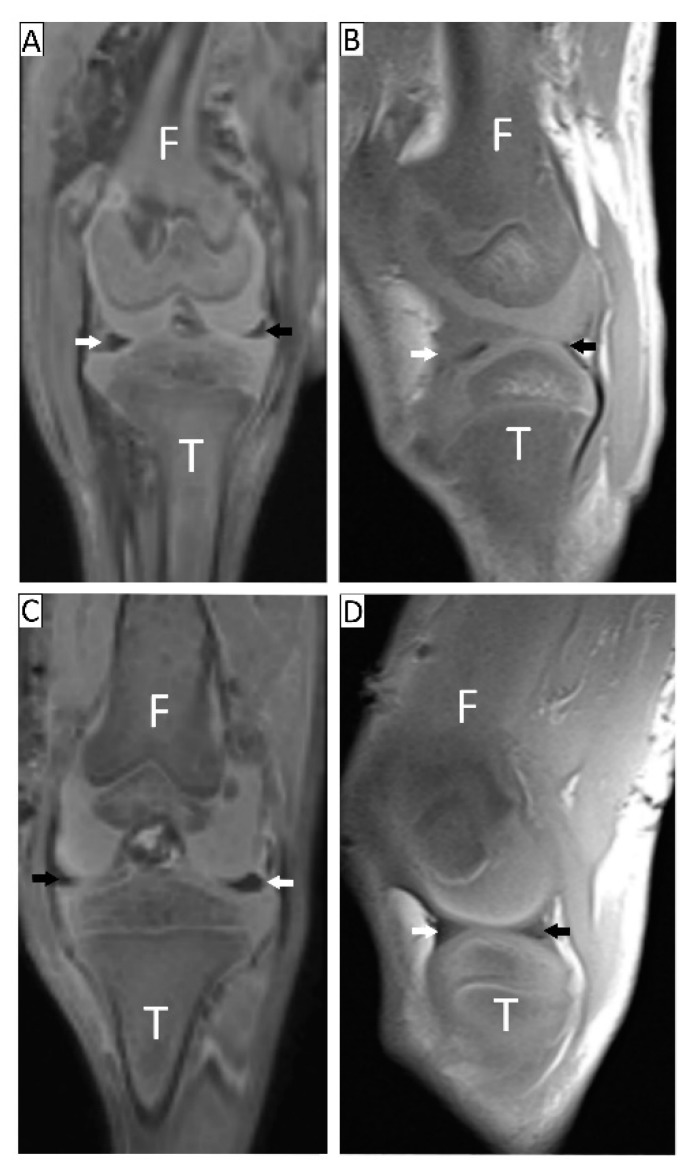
MR acquisition of a quadriceps-contracture-syndrome-affected knee and a healthy one. Coronal (A,C) and sagittal (B,D) reformat of 3D T1-weighted MR acquisition. A,B: right contracted limb; C,D: left noncontracted limb. The different morphology of menisci is clearly seen (white arrow, lateral meniscus body; black arrow, medial meniscus body; F, femur; T, tibia).
